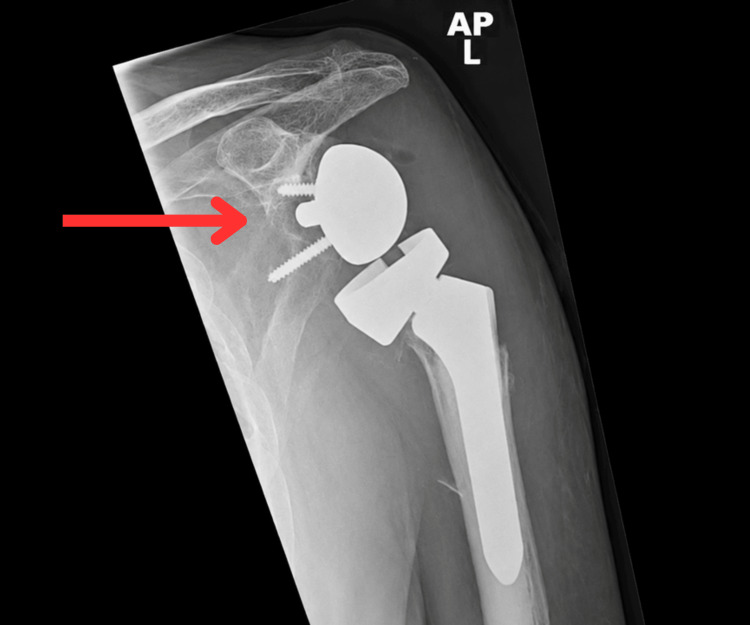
Post-operative radiograph following the first open revision
